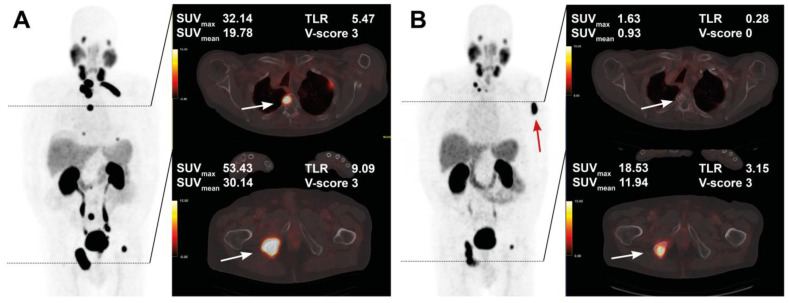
Pre- and post-treatment images in an 83-year-old patient after 5 cycles of RLT with cumulative 35.1 GBq [^177^Lu]Lu-PSMA-617. Maximum intensity projection (MIP) images (left) with cross-section fusion images in a thoracic and pelvic plane at baseline (A) and after RLT (B). Lesions (white arrows) with intense baseline uptake showing response to RLT; a new bone metastasis develops in the left humerus (red arrow).
